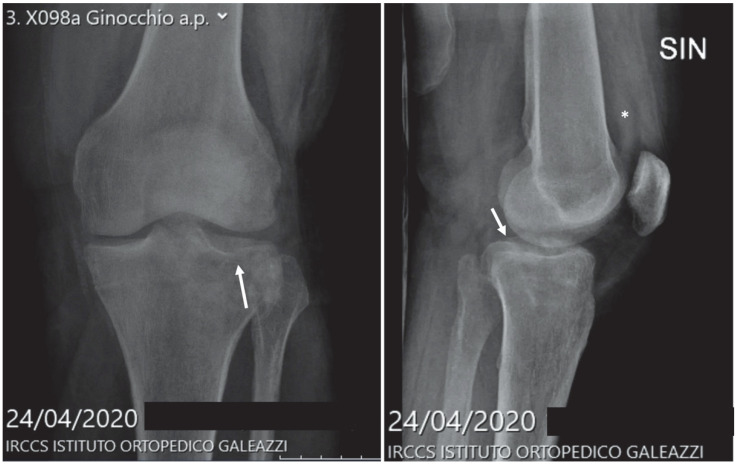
Figure shows a frontal and lateral knee X-ray examination of a 67-year-old male patient who suffered a non-displaced external left tibial plateau fracture (arrow) that has been treated conservatively with home-discharge after immobilization. Lateral X-ray shows mild knee post-traumatic effusion (asterisk).
